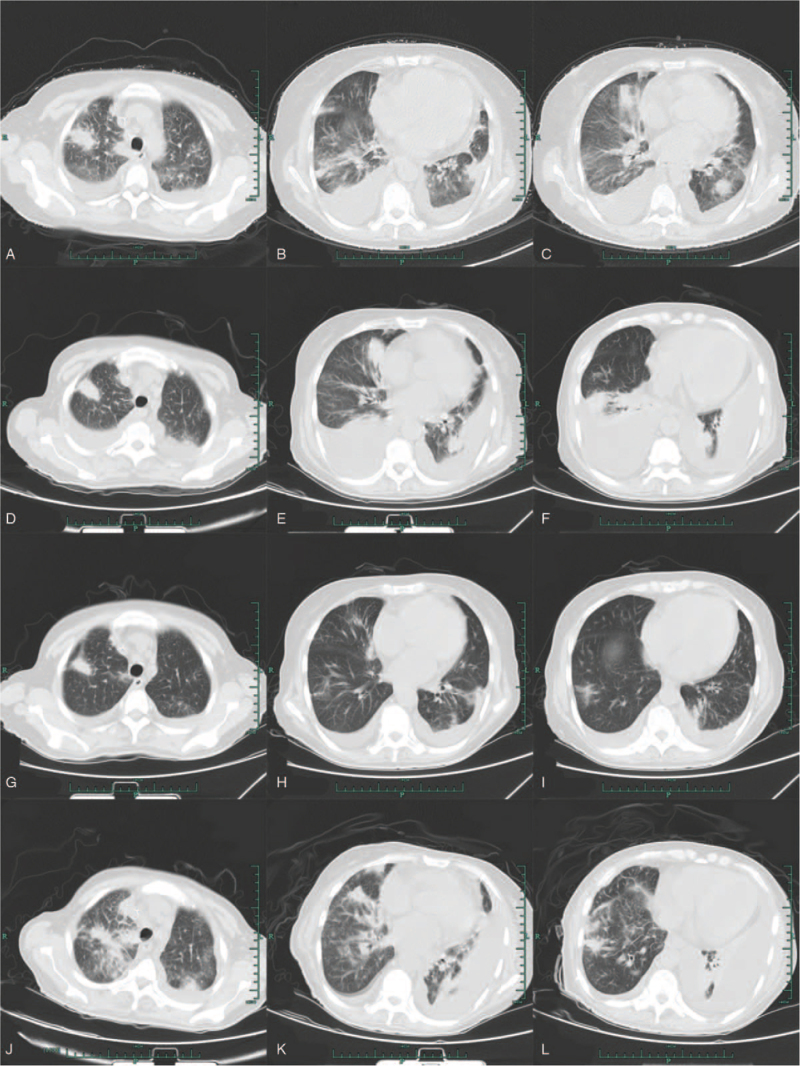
Chest CT scanning showing the metastatic lymphangitis carcinomatosa in lungs. A–C. April 12, 2020; D–F. May 12, 2020; G–I. May 19, 2020; J–L. July 9, 2020. CT = computer tomography.
